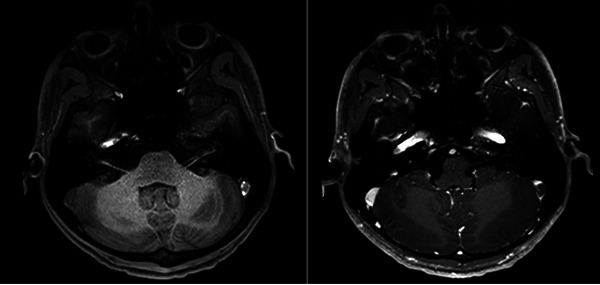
Brain MRI showing left sigmoid sinus thrombosis. (A) Axial T1 scan showing spontaneous hyperintensity in the left sigmoid sinus indicating the thrombus. (B) Axial T1 scan after gadolinium injection showing an endoluminal defect in the left sigmoid sinus indicating thrombosis.
